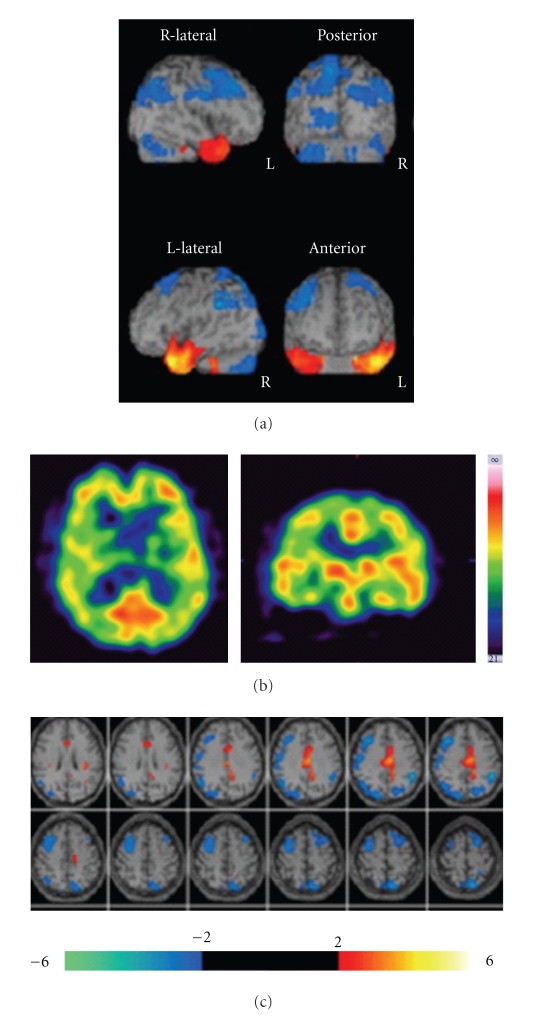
Brain ^99m^Tc-ECD SPECT imaging before lomerizine treatment, CBF is decreased in bilateral frontoparietal and the left occipital regions on eZIS imaging.
